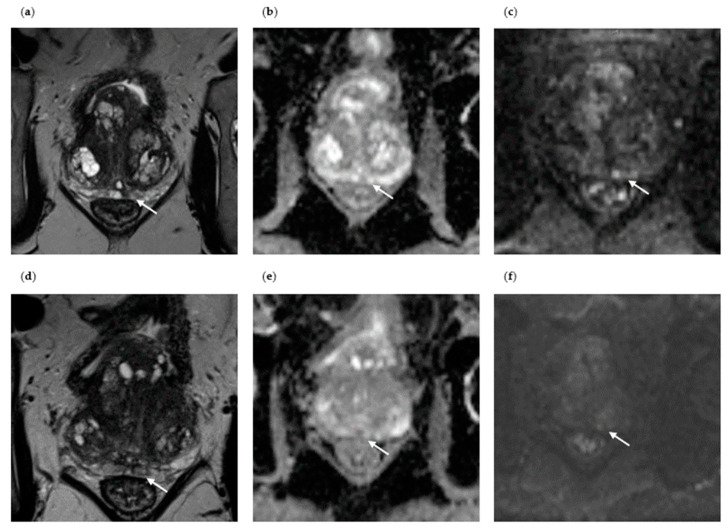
Axial mpMRI of a patient without PC detection at initial and repeat biopsy (Patient III). (a) Initial mpMRI of Patient III without PC detection at initial biopsy (PI-RADS lesion 4, T2-weighted image, the suspicious lesion is marked with an arrow); (b) marked ADC reduction in the PI-RADS 4 lesion at initial biopsy; (c) marked signal enhancement of the PI-RADS 4 lesion in the high b-value image at initial biopsy; (d) repeat mpMRI of Patient III without PC detection at repeat biopsy (PI-RADS lesion 3, T2-weighted image, focal lesion regressive T2 hypointense); (e) flat ADC reduction in the PI-RADS 3 lesion at repeat biopsy; (f) signal enhancement of the PI-RADS 3 lesion in the high b-value image at repeat biopsy.
